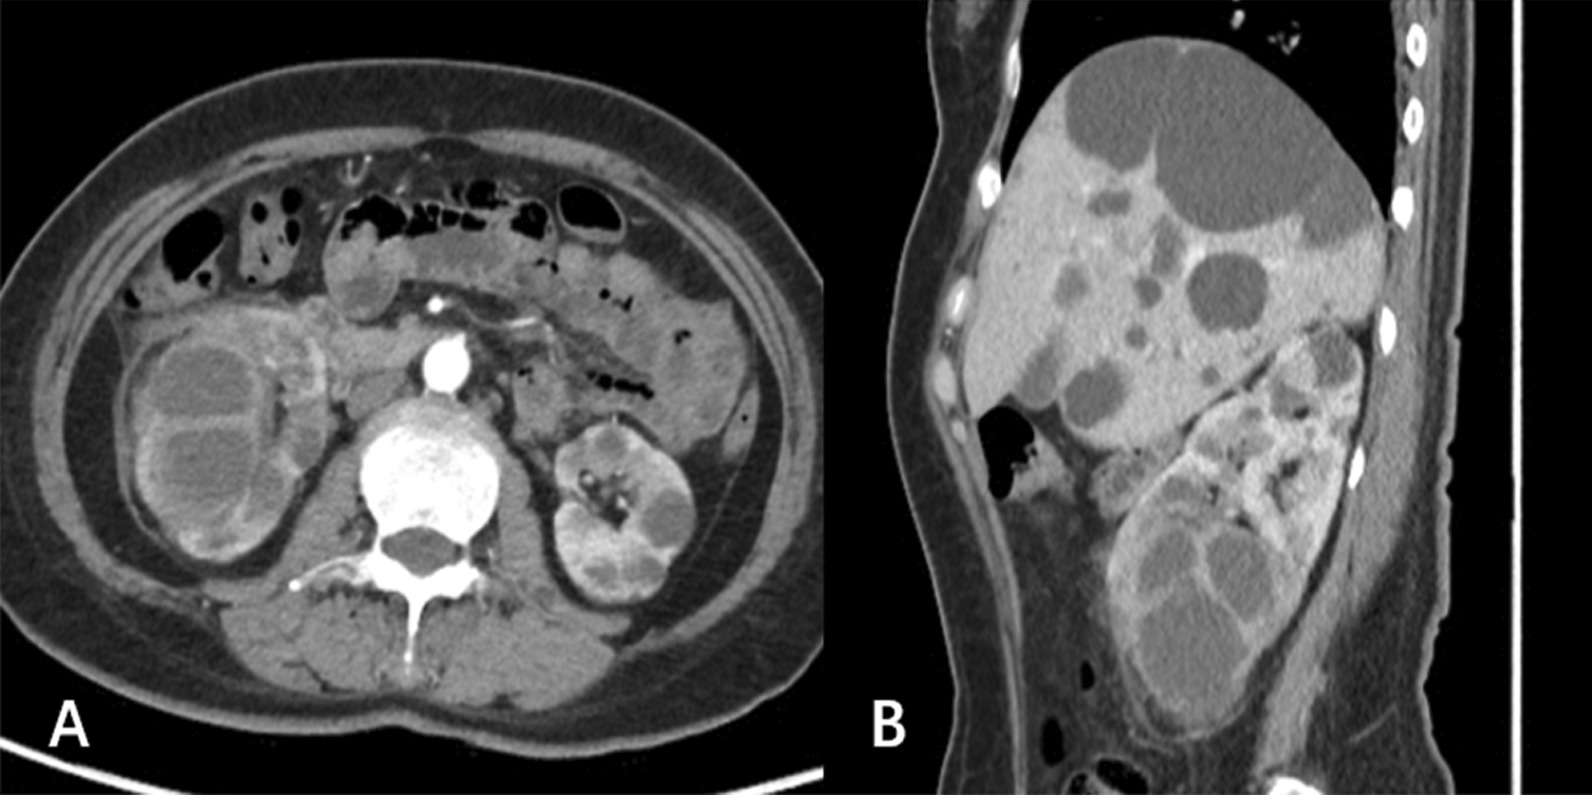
A axial and B sagittal contrast-enhanced CT images of the middle and the lower abdomen
